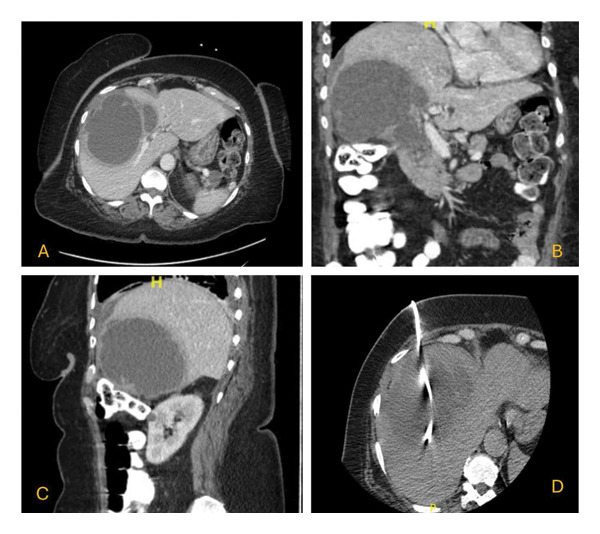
Contrast‐enhanced computed tomography images of the abdomen: (A) axial, (B) coronal, and (C) sagittal views revealing grossly distended gall bladder with wall thickening, pericholecystic fluid, and edema of the adjacent hepatic parenchyma. (D) Axial image following percutaneous image–guided cholecystostomy placement.
